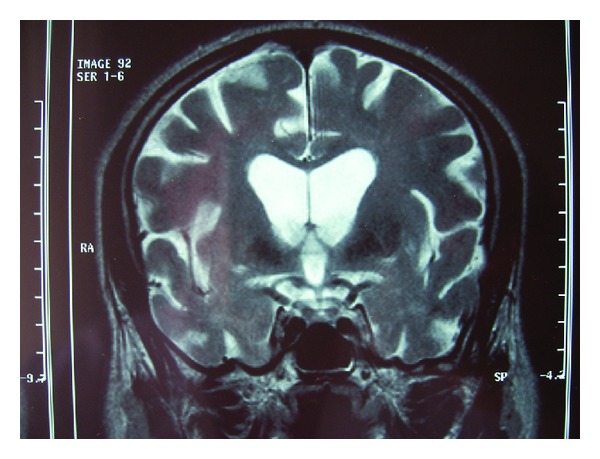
Bilateral calcifications in the basal ganglia and white matter signal changes on MRI.
